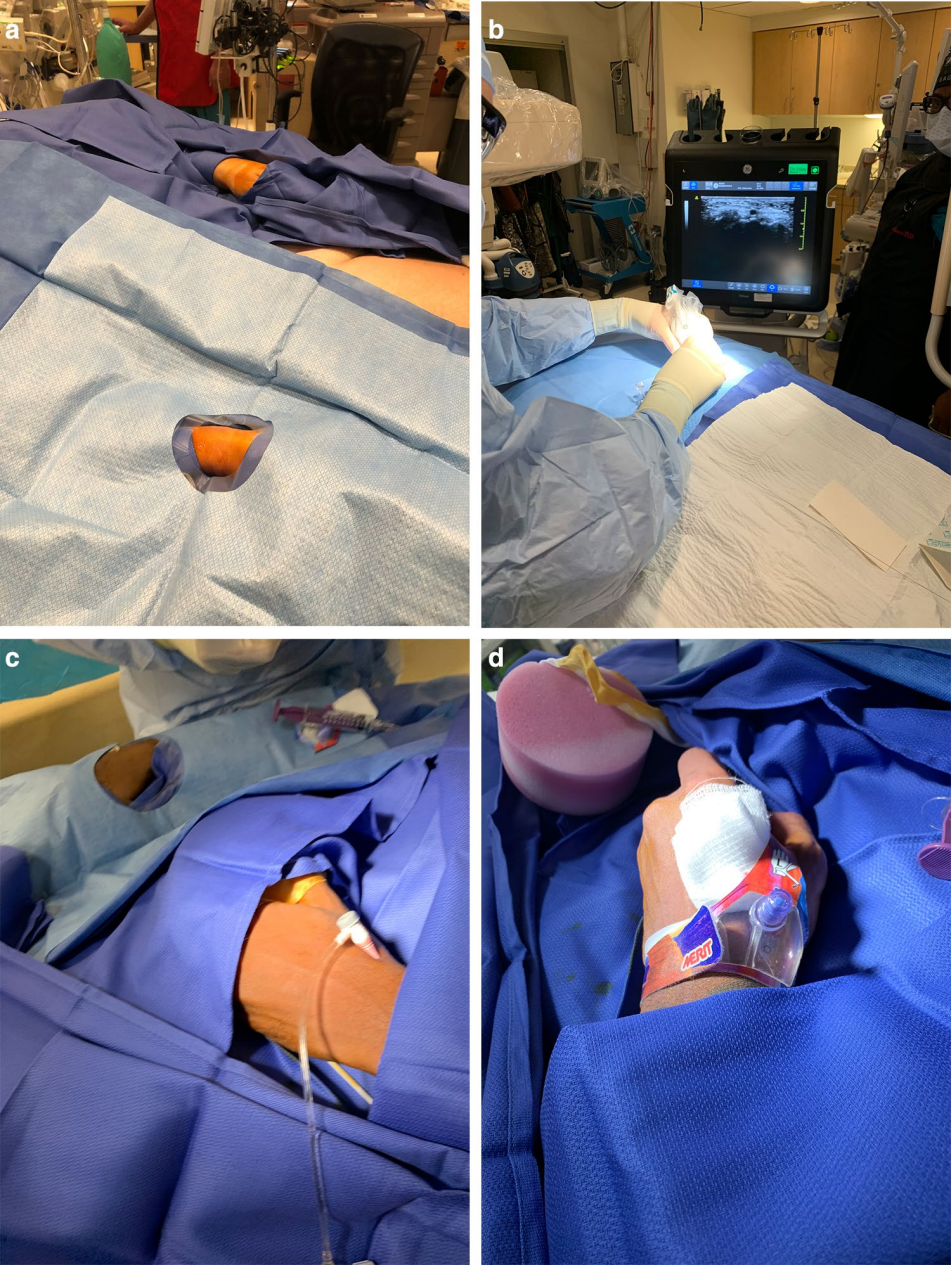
Pictures depicting our standard setup for left transradial neuroangiography. (a) Note the position of the left hand over the left groin, and the right wrist is prepped and draped as well. (b) Ultrasound guidance is utilized. (c) The sheath in inserted through the distal transradial artery “Snuff box approach.” (d) Closure is performed with the Prelude Sync radial compression device.
